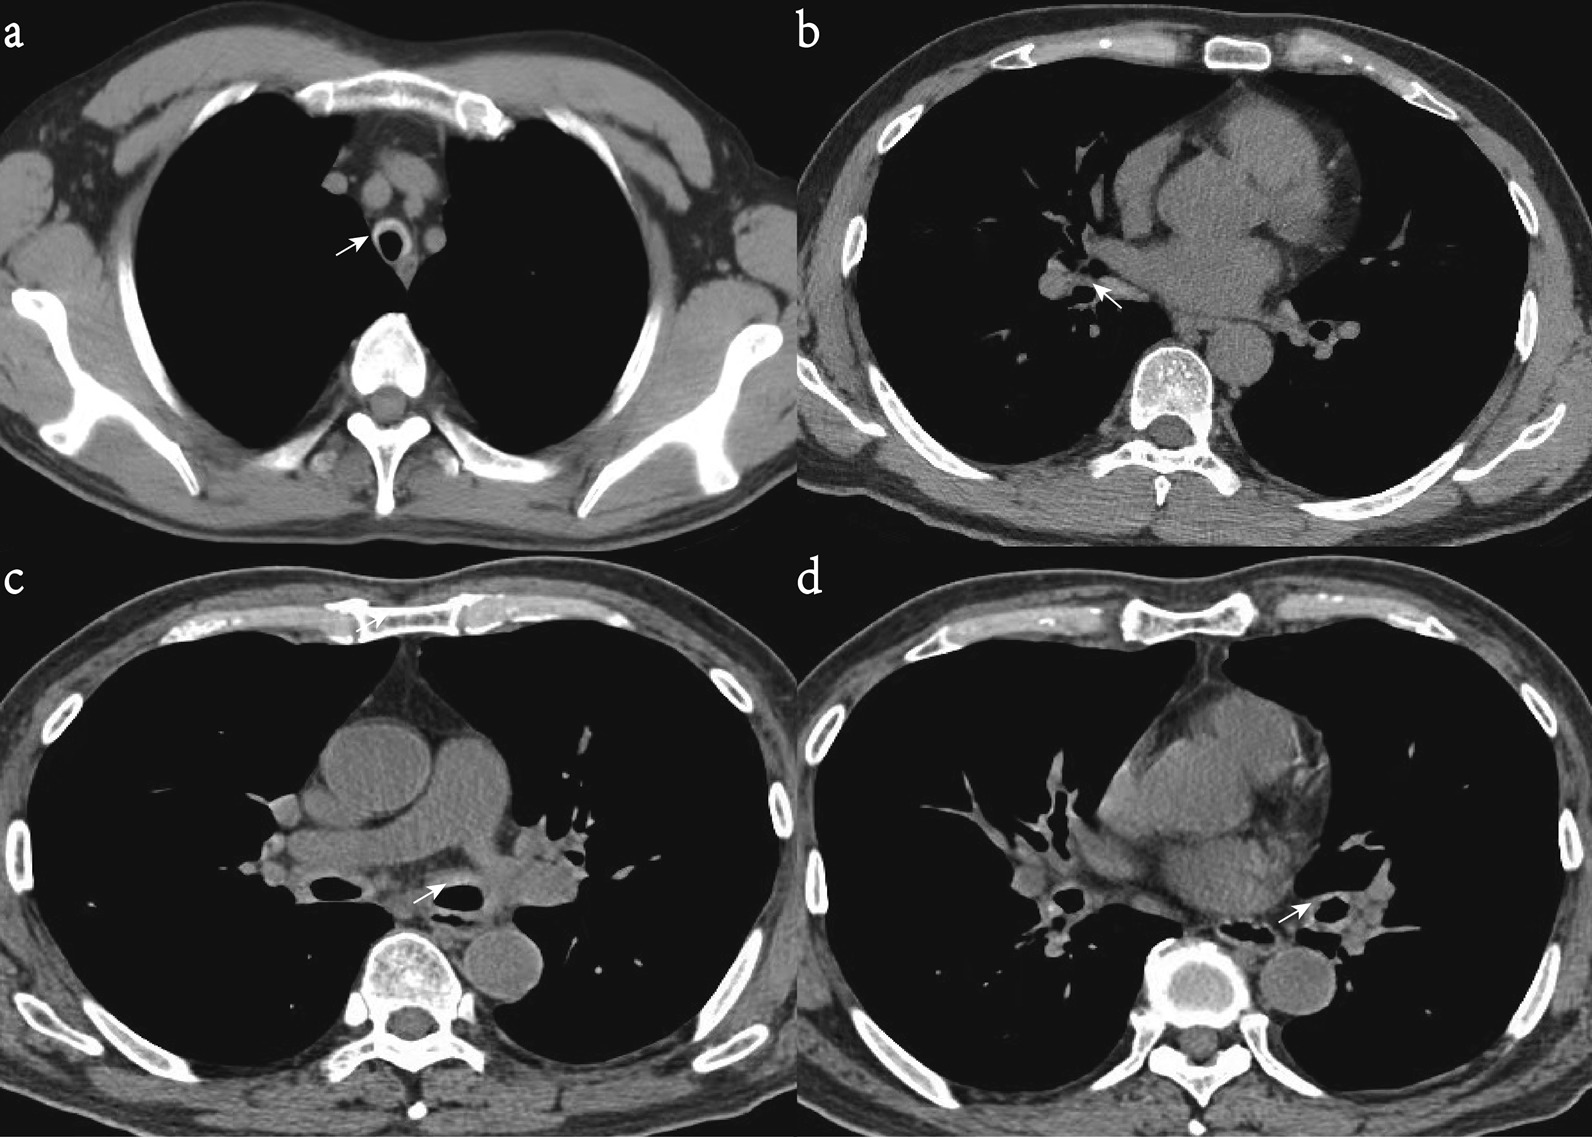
Axial CT slices showing thickening of the anterior and bilateral wall (arrow) of the upper trachea (a) in a 36-year-old woman with relapsing polychondritis. Axial CT slices showing thickening of the anterior and bilateral wall (arrow) of right inferior lobar bronchus (b), left main bronchus (c), left inferior lobar bronchus (d) in a 41-year-old man with relapsing polychondritis
